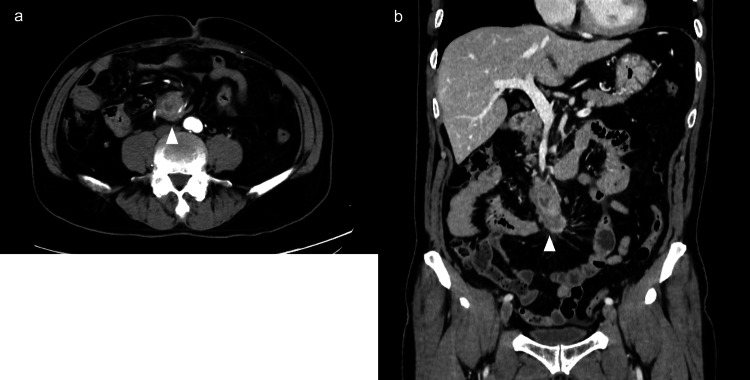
Contrast-enhanced CT images Contrast-enhanced CT imaging showing an irregularly shaped mass measuring 30 × 50 mm in the mesentery near the duodenum, along with several small lymph nodes in the surrounding area (a: axial view; b: coronal view). CT, computed tomography
